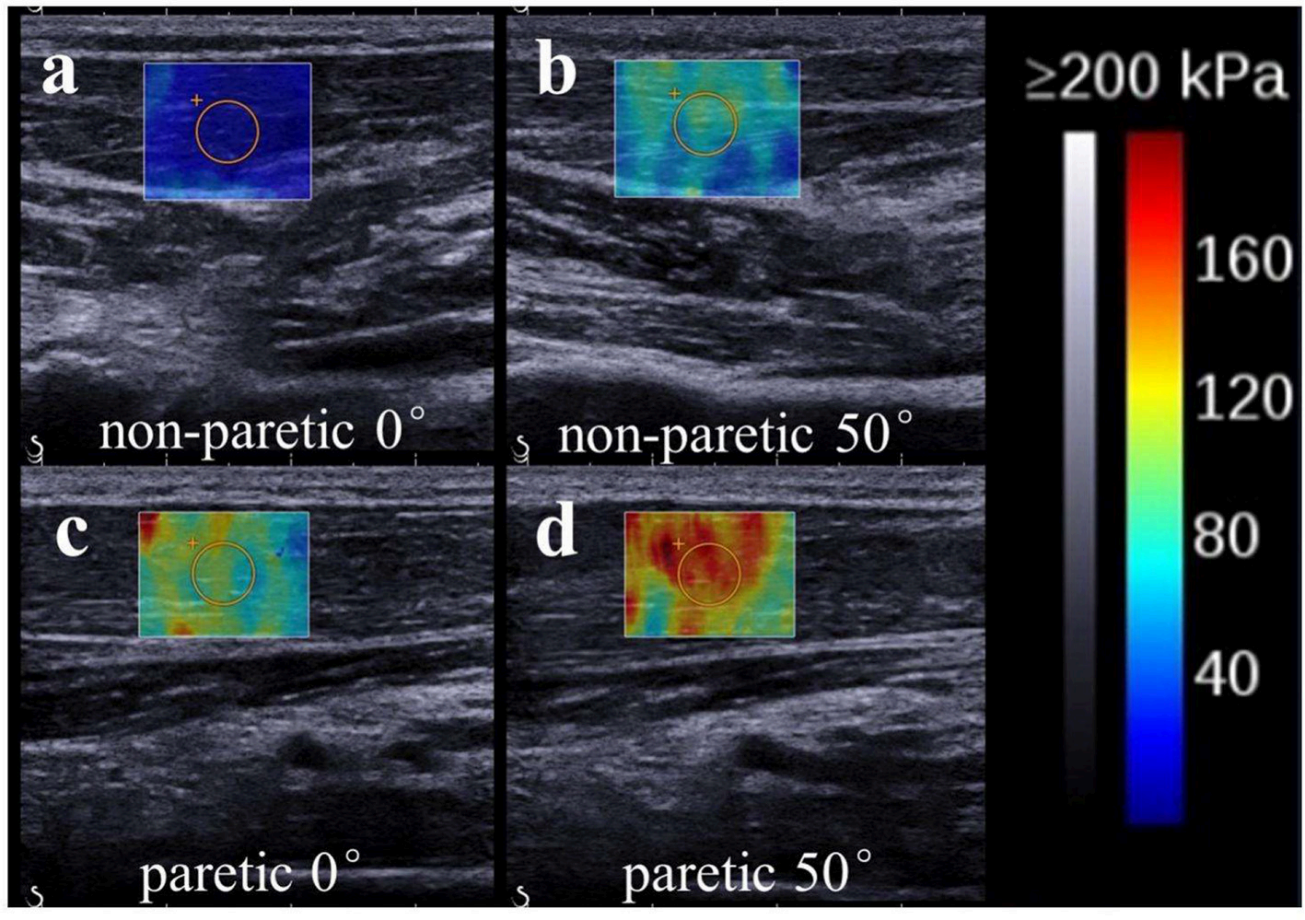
Representative ultrasound images (SWE ultrasound map superimposed on the B-mode image) of the non-paretic (a:0°; b:50°) and paretic (c:0°; d:50°) flexor carpi radialis. The value of shear wave modulus is greater in the paretic limb. We found the non-paretic muscle has a lower value of shear elastic modulus than the paretic muscle at the same wrist angle. Meanwhile, non-paretic and paretic muscle both has a lower value of shear elastic modulus when wrist was held at 0° than wrist was passive stretched at 50°.
